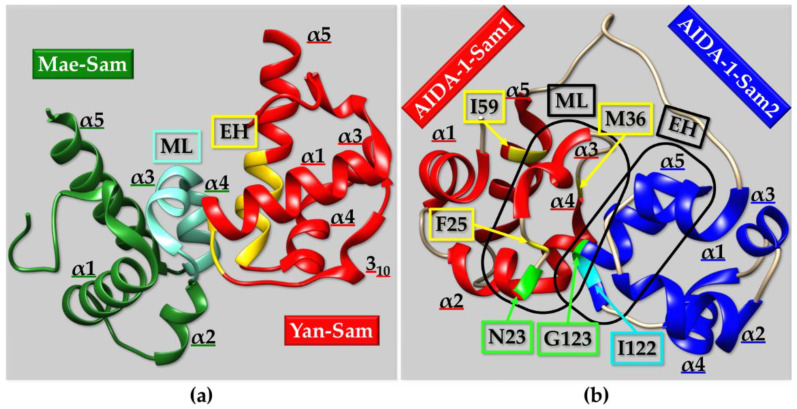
(a) X-ray structure of the Yan-Sam (A86R mutant) (red)/Mae-Sam (green) complex (PDB entry 1SV0 [78], chains A and C). The regions defining the EH interface in Yan-Sam are colored yellow, whereas those encompassing the ML interface in Mae-Sam are highlighted in cyan. (b) NMR structure of the AIDA-1 tandem Sam1 and Sam2 domains (PDB entry 2KIV [89], conformer n.1). A few crucial residues contributing to the hydrophobic binding surface are indicated in yellow if belonging to Sam1 (i.e., F25, M36 and I59) and in cyan if belonging to Sam2 (i.e., I122). The residues N23 (from Sam1 ML surface) and G123 (from Sam2 EH region), whose backbone _C_O and H_N_, respectively form a characteristic hydrogen bond at the Sam1-Sam2 interface are colored in green.
